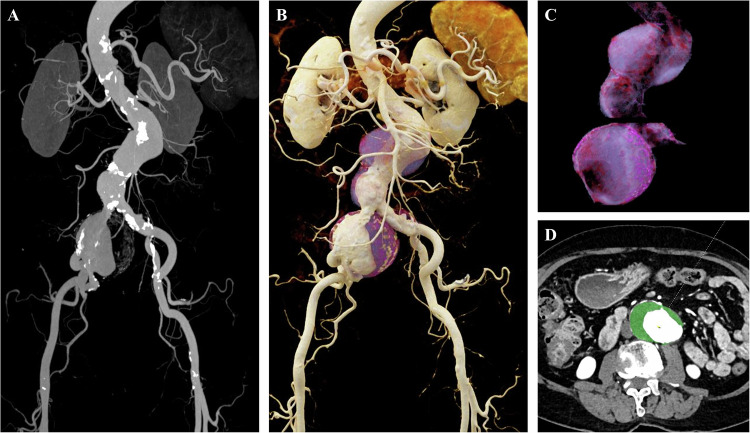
Abdominal aortic aneurysm in an 87-year-old patient. A The CTA-based MIP image reveals varying degrees of luminal dilation and stenosis in the distal abdominal aorta and right common iliac artery; however, the extent of the mural thrombus is challenging to intuitively visualize. B The CTA-based CR image vividly delineates the overall morphology of the aneurysm and the volumetric distribution of the mural thrombus (highlighted in purple). C, D CR reconstructions of the extracted mural thrombus (highlighted in purple in C, and green in D), segmented based on the region of interest, facilitate precise volumetric quantification and subsequent compositional analysis
